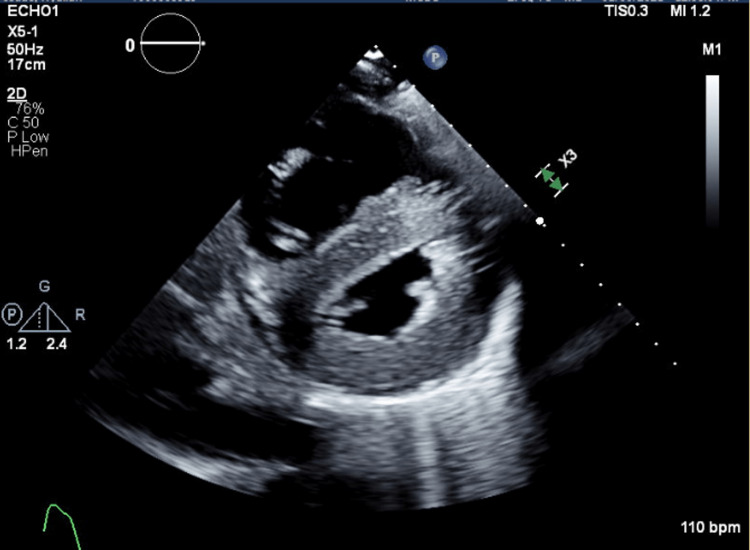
Parasternal Short Axis View - Transthoracic Echocardiogram
